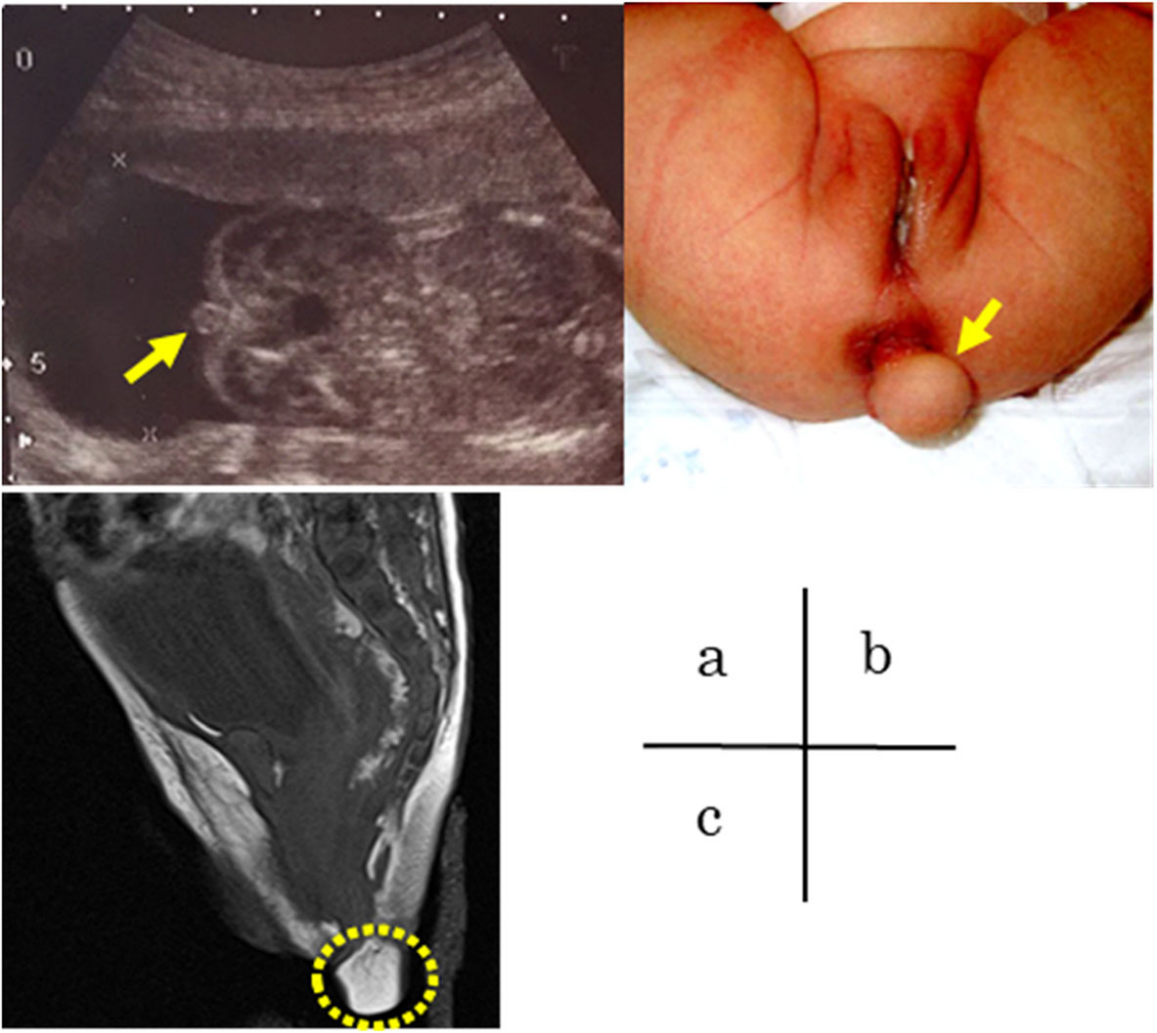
a Prenatal ultrasound showing the perineal lipoma (arrow) at gestational age of 20 weeks and 4 days. b Postnatal appearance of the soft round mass just left of the anal verge (arrow). c T2-weighted magnetic resonance imaging (sagittal view) of the perianal mass (dotted circle) in indicating a signal intensity consistent with fat located close to anus, and no spinal anomaly
